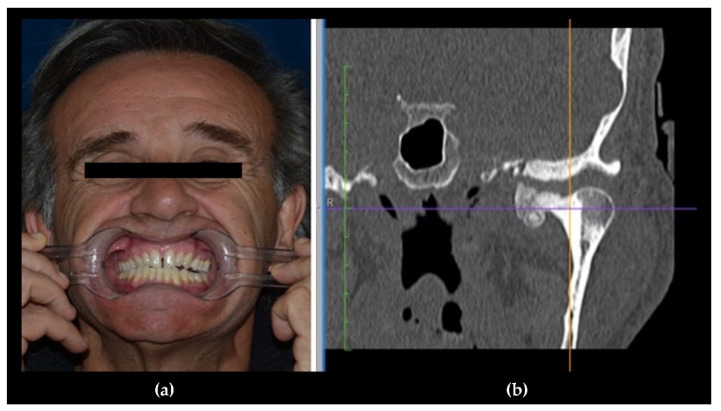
(a) A male patient with dental malocclusion, characterized by left open bite and right cross-bite; (b) CT scan shows a left condylar osteoma.
